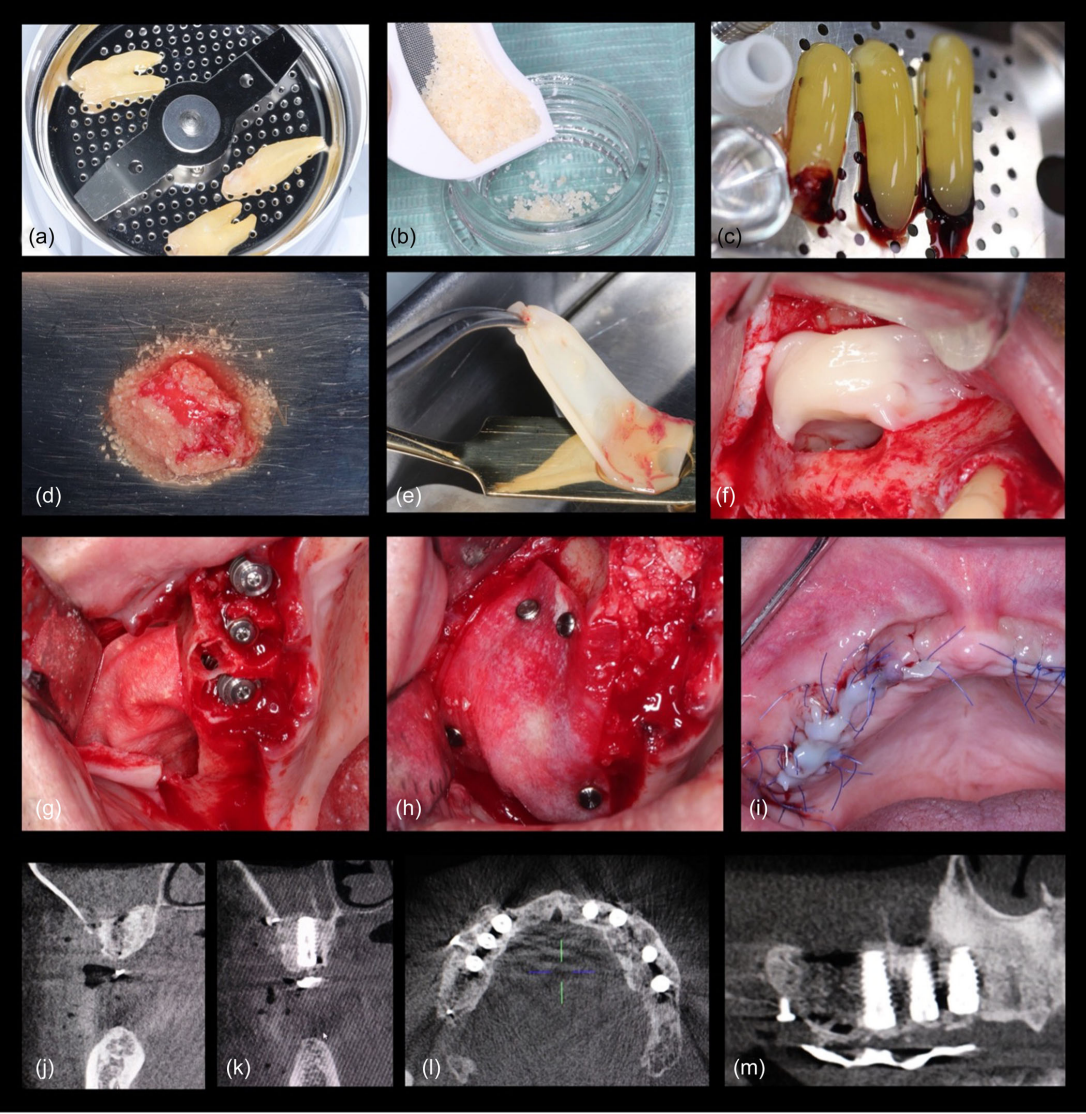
Showcases the following steps of the procedure: (a) Extracted teeth are placed in the grinding machine. (b) The resulting dentin graft with particle sizes ranging from 250 to 1000 μm. (c) Platelet‐rich fibrin (PRF) was prepared. (d) The dentin graft is mixed with the liquid form of PRF. (e, f) PRF membrane was utilized to cover the sinus membrane before placing the dentin graft. (g, h) Collagen membrane employed for intra and extra‐sinus applications. (i) Flap closure. (j–m) Various cone beam computed tomography views captured after sinus augmentation.
